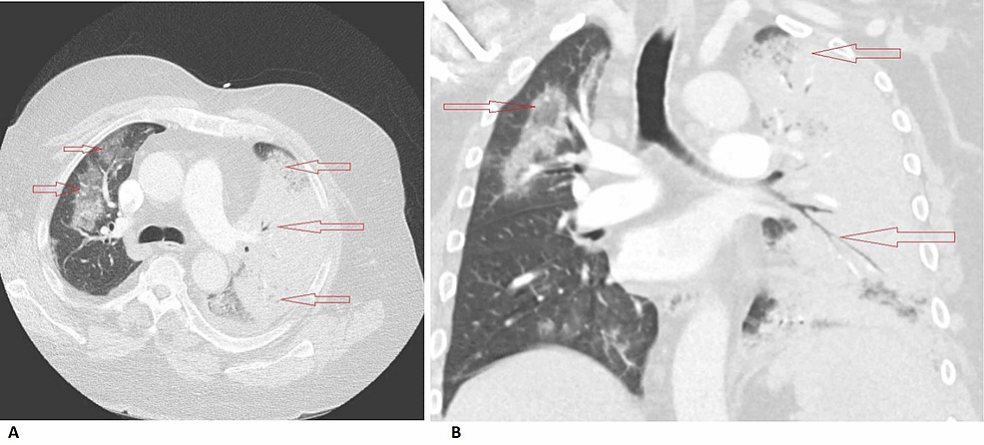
Chest CT demonstrates complete opacification of the left lung fields with diffuse pneumonia, trace pleural effusion, and multiple ground glass alveolar infiltrates involving the right upper, middle, and lower lobes in (A) transverse view and (B) coronal view.
